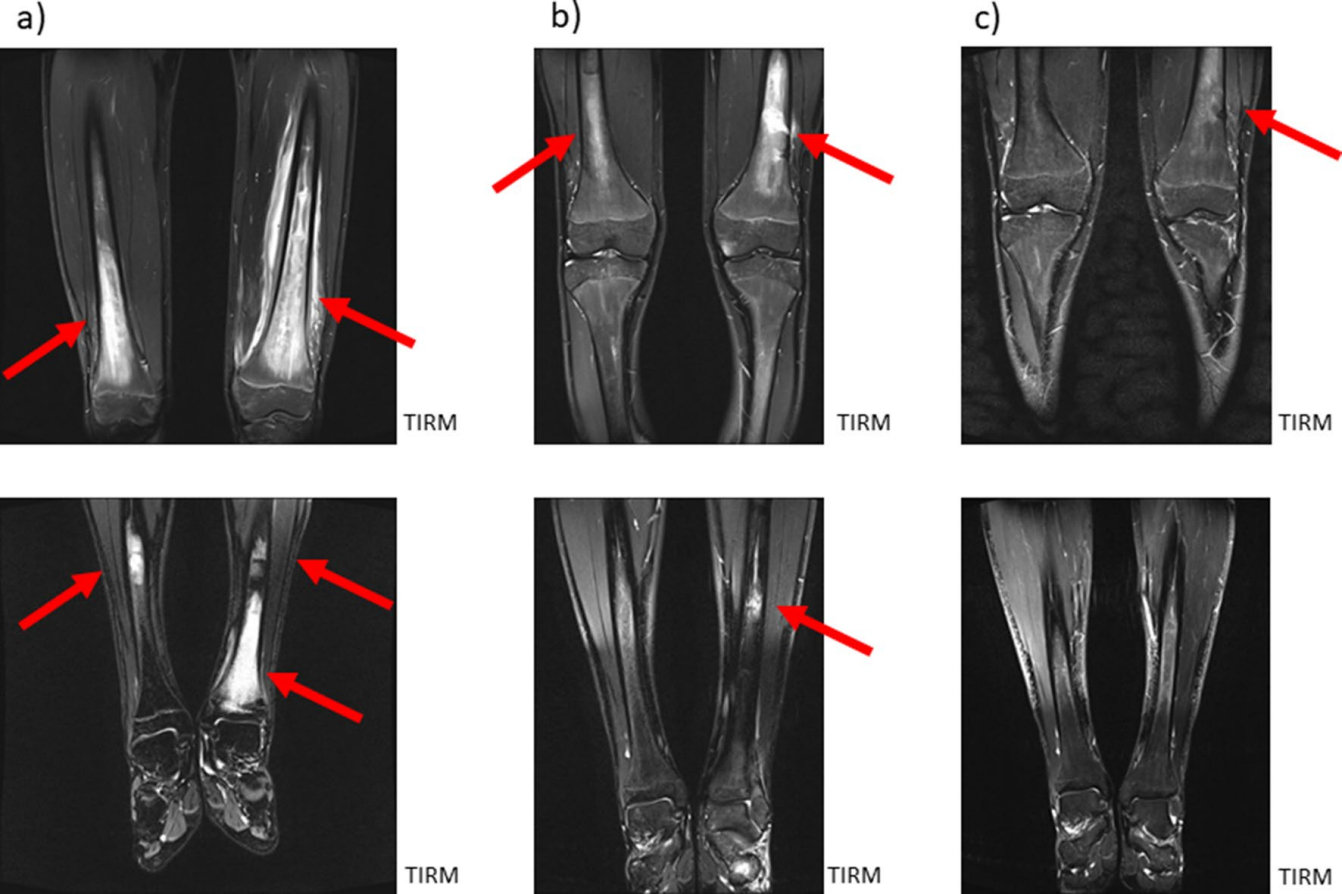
A 16-year-old adolescent with exhaustion for 2 months, bone and muscle pain. MRI showed extensive bone marrow edema in the distal femora and tibiae, marked concomitant soft tissue reaction within the left femur and less marked in the left tibia. After bone biopsy (femur) and diagnosis of CNO, initial therapy with naproxen yields clinical regression. MRI demonstrated regression. Under naproxen therapy asymptomatic patient with still visible lesions
